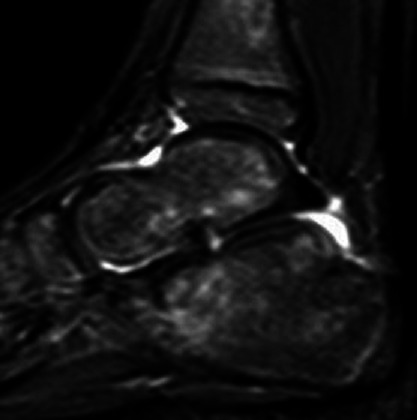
Repeat T2 weight sequence MRI showing resolution of marrow oedema. Cavity left by curettage and filled with bone graft.
